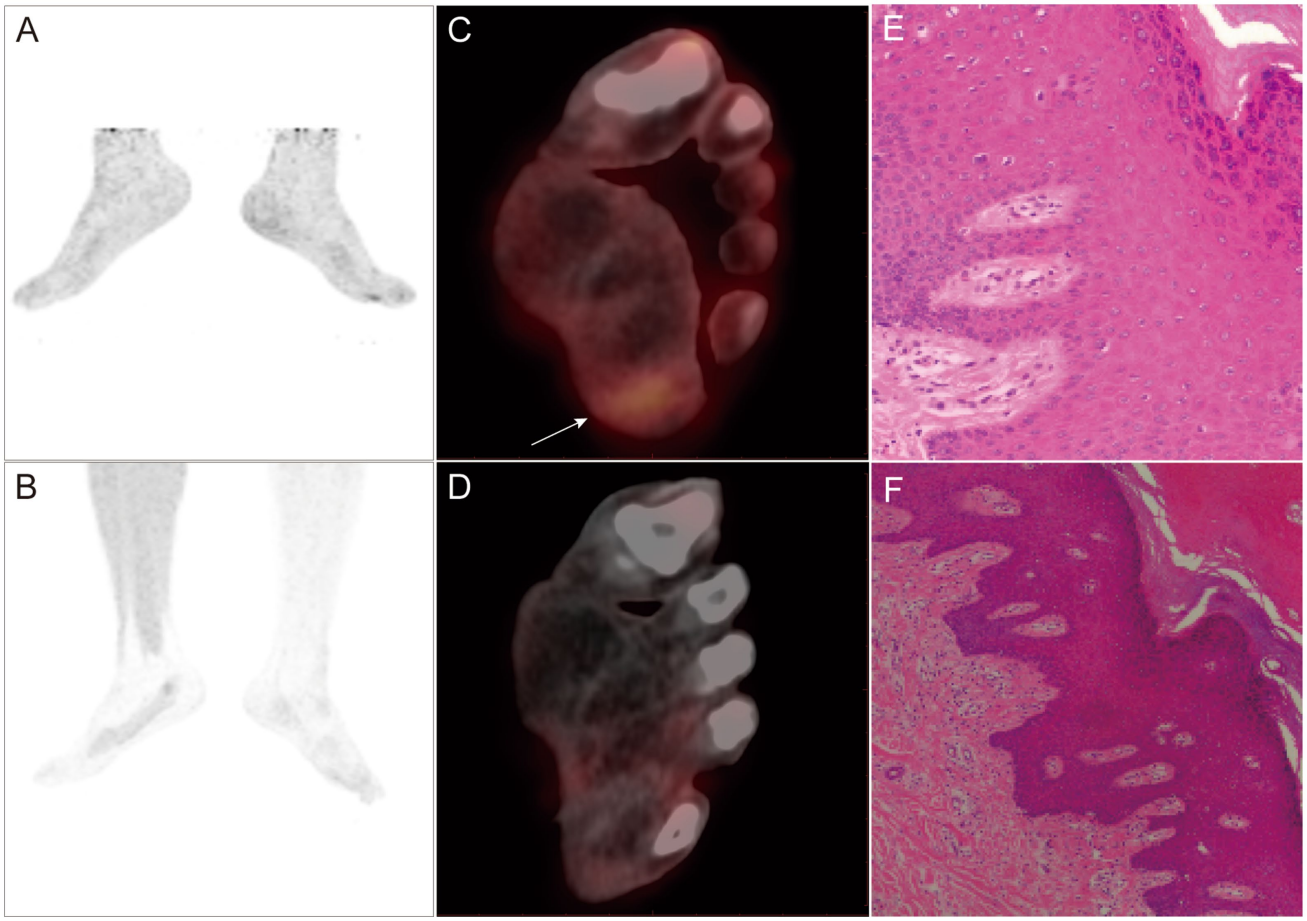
A-F PET/CT and pathological images of a 35-year-old male patient with melanoma who underwent plantar lesion resection surgery 3 weeks before the study took place. ^18^F-FDG PET/CT MIP (A) and PET/CT (C) showing local radioactive accumulation shadow in the left sole (SUVmax: 2.7). ^18^F-P3BZA PET/CT MIP (B) and PET/CT (D) without any abnormal radioactive uptake. Five days later, an extended resection of the surgical area was performed, and postoperative pathology confirmed no evidence of tumor recurrence (active local squamous cell proliferation, E,F).
